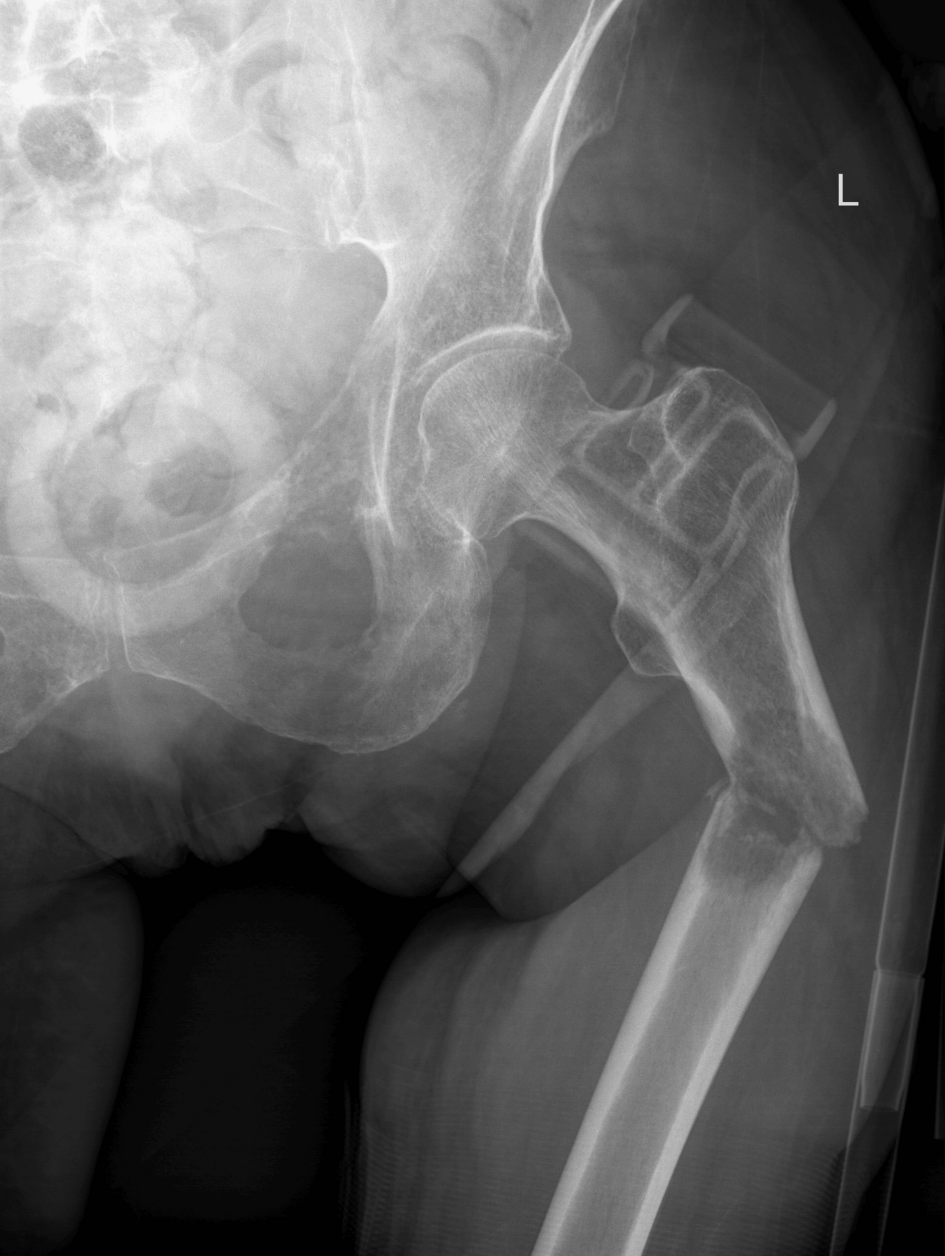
X-ray of the right hip, anteroposterior view, showing a left femoral shaft fracture
